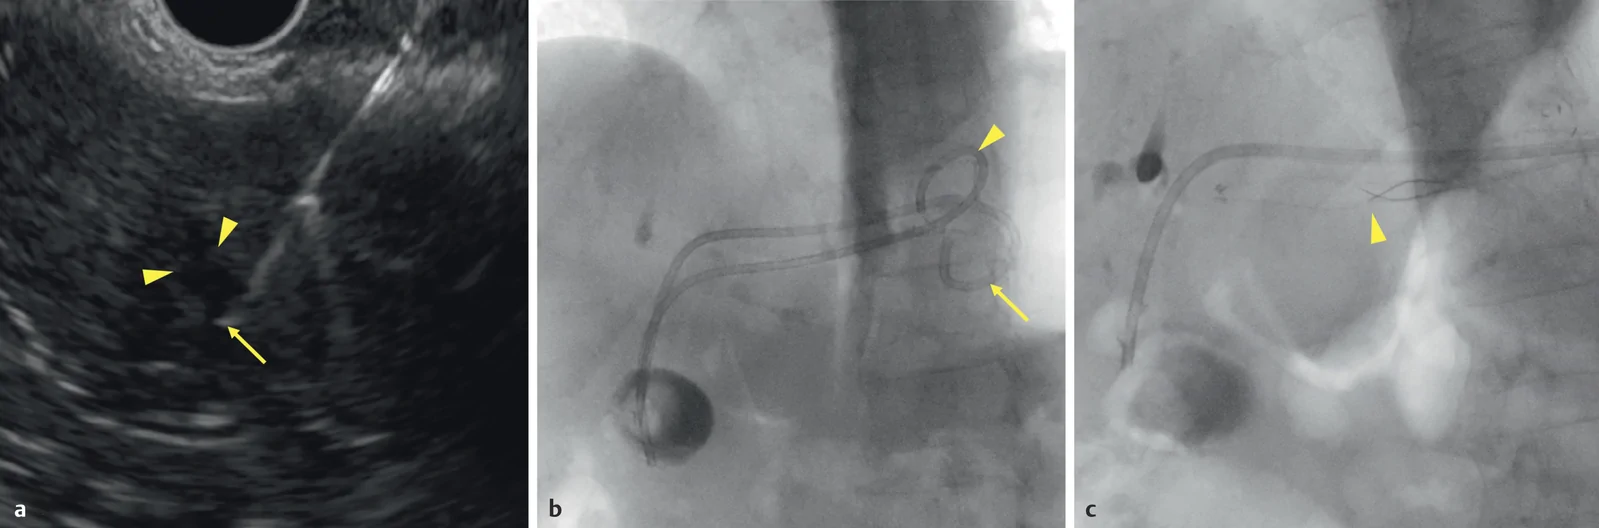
Images obtained during endoscopic ultrasound–guided
